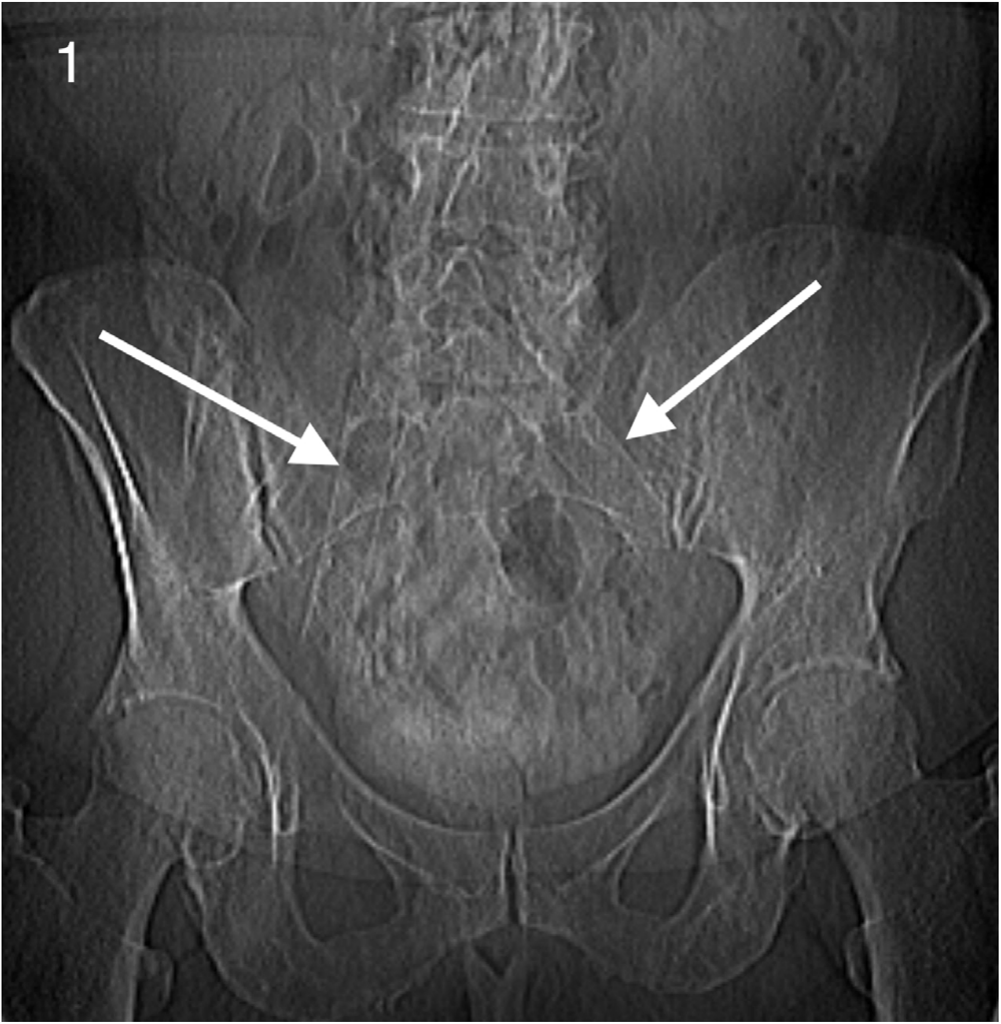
Scout view radiograph demonstrating bilateral common iliac vein stents placed for May-Thurner Syndrome (white arrows).
